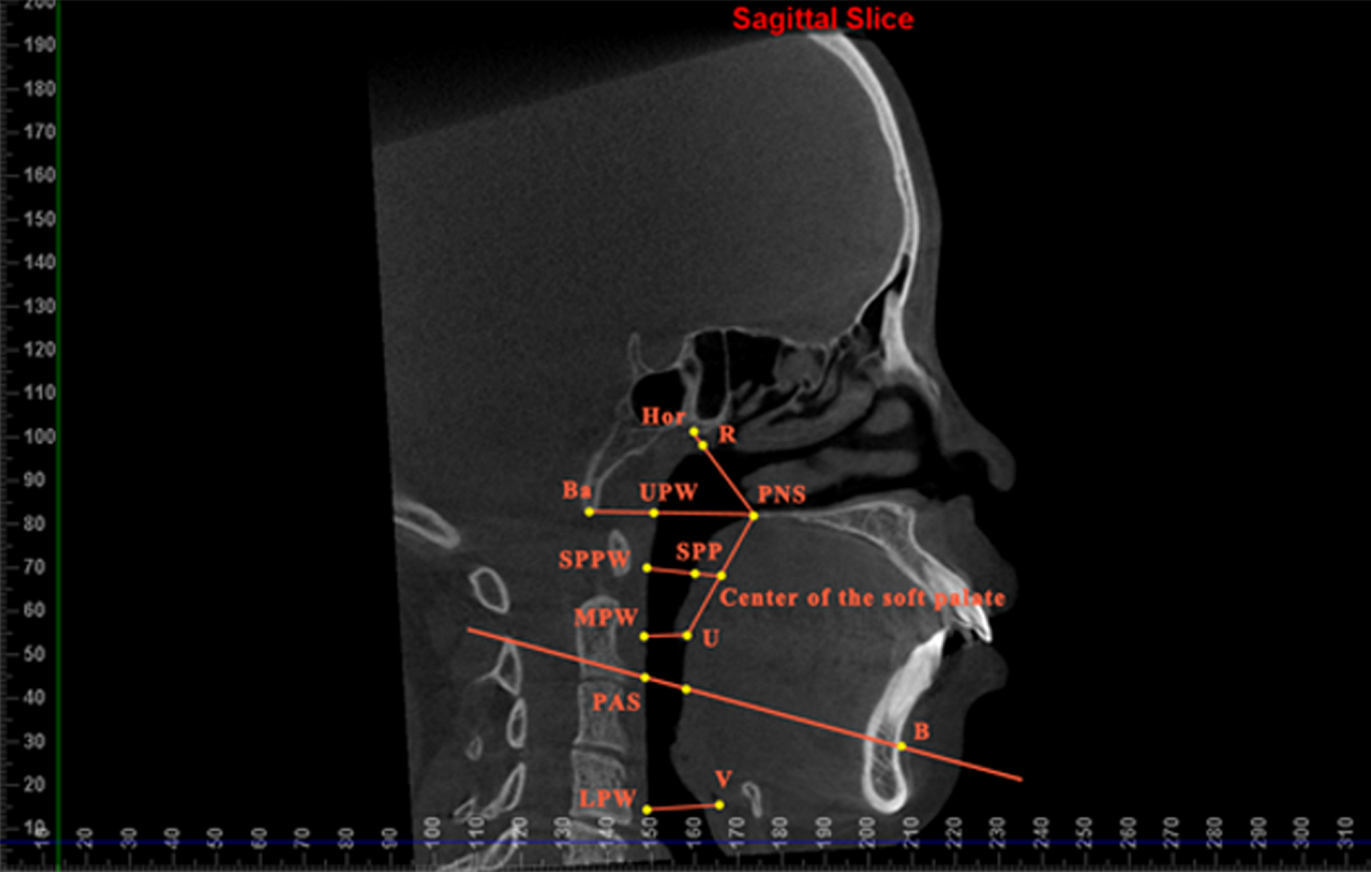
Measurements of the sagittal airway dimension in the mid-sagittal plane of CBCT.
